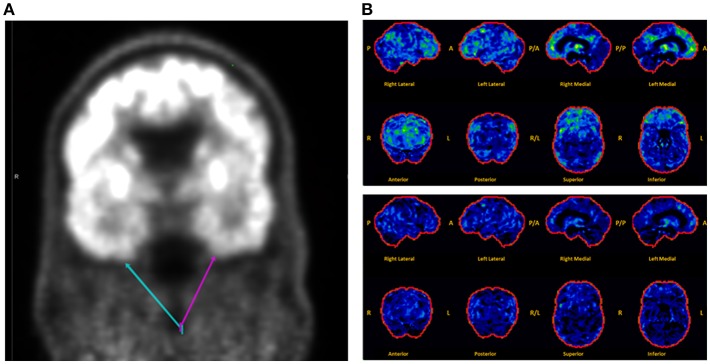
Montage of abnormal brain FDG-PET scans in a case series of transient epileptic amnesia. Representative images are displayed for two individuals meeting diagnostic criteria for TEA who displayed focal hypometabolism on FDG-PET. (A) This 75-year-old man was evaluated for 6 months of amnestic spells, irritability, and disinhibition, and displayed subtle focal hypometabolism in the anteroinferior aspect of the left temporal lobe (magenta arrow) as compared to the right (teal arrow). (B) This 71-year-old man presented for evaluation of longstanding personality changes (starting at age 57) and more recent recurrent spells of amnesia and confusion, and displayed normal brain MRI and left frontal and right frontotemporal epileptiform abnormalities on EEG during sleep. (Top Panel) Brain FDG-PET prior to initiation of antiepileptic therapy showed hypometabolism (indicated by regions in green and yellow) most marked in the left medial frontal, anterior cingulate, and medial temporal regions. (Bottom Panel) Brain FDG-PET 1 year after initiation of lamotrigine showed extensive resolution of the previously visualized hypometabolism. The patient clinically improved during that time, with resolution of both the personality changes and spells. A, anterior; P, posterior; R, right; L, left.
